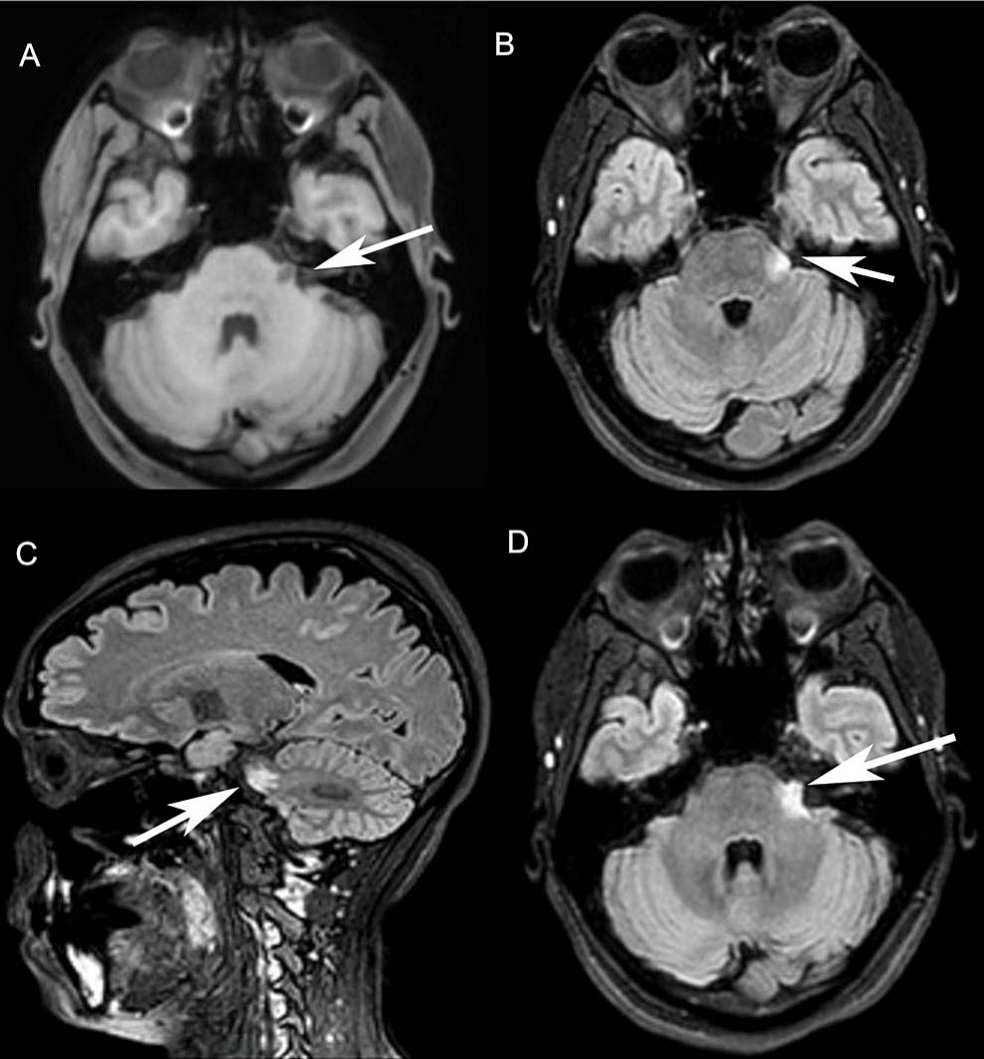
(A) T1-weighted image: Axial plane showing a hypo-intense small intraparenchymal lesion measuring 7 mm in anteroposterior diameter at the level of the nuclei of the left trigeminal nerve present at the junction between the pons and left brachium pontis. (B) FLAIR weighted sequence: Axial plane showing a wider hyper-intense intraparenchymal lesion measuring 14 mm in anteroposterior diameter and 7 mm in thickness at the level of the nuclei of the left trigeminal nerve present at the junction between the pons and left brachium pontis, appearing larger than its T1 counterpart. (C) FLAIR weighted sequence: Sagittal plane showing the hyper-intense pontine intraparenchymal lesion measuring 9 mm in superoinferior diameter. (D) FLAIR weighted sequence: Axial plane showing a hyper-intense small extraparenchymal lesion at the level of the nuclei of the left trigeminal nerve at the junction between the pons and left brachium pontis, measuring 4 mm extending along the left trigeminal nerve that is thickened in its preganglionic portion before the left Meckel’s cave in addition to the intraparenchymal lesion. FLAIR: Fluid-attenuated inversion recovery
